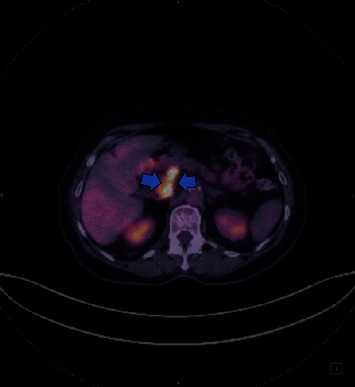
PET/CT transverse image: an area of linearly increased metabolic activity is observed in the upper abdomen, adjacent to the pancreas, which corresponds to a part of the portal vein and superior mesenteric vein consistent with thrombosis (neoplastic thrombosis) (blue arrows).
